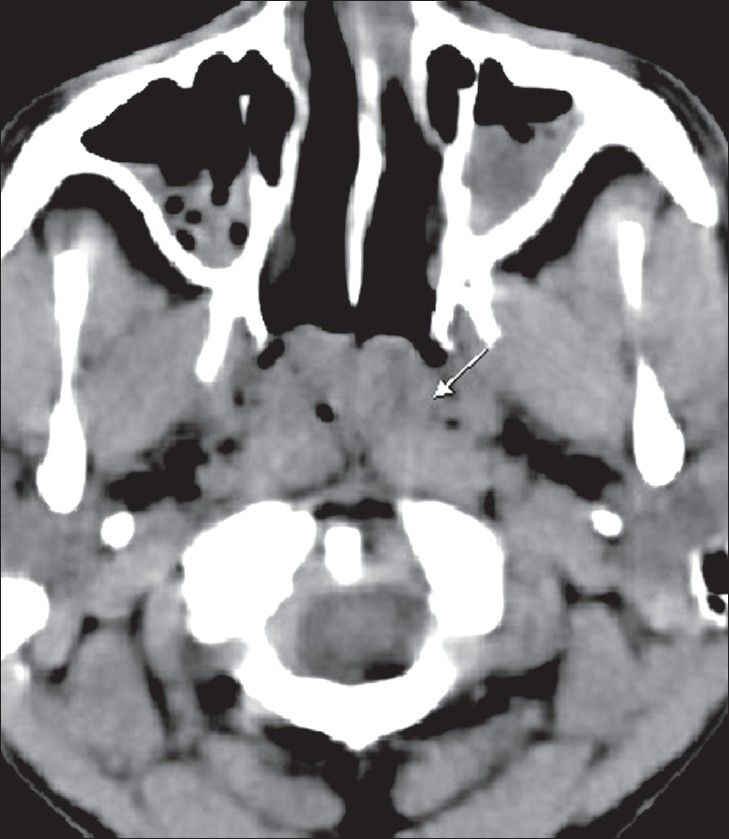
Plain CT scan image shows a soft tissue mass lesion in the roof of the nasopharynx (arrow)
